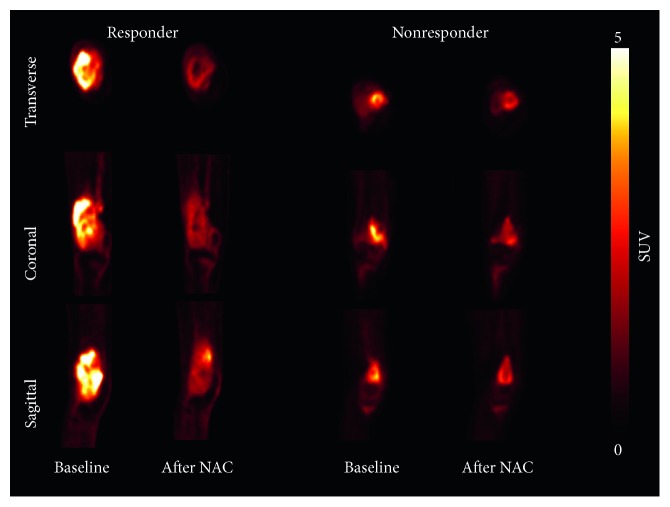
Representative ^18^F-FDG PET images of a responder and a nonresponder with osteosarcoma. Responder SUVmax values were 11.33 and 4.43 at baseline and after neoadjuvant chemotherapy (NAC), respectively. Nonresponders had SUVmax values of 5.62 and 3.21 at baseline and after NAC, respectively.
